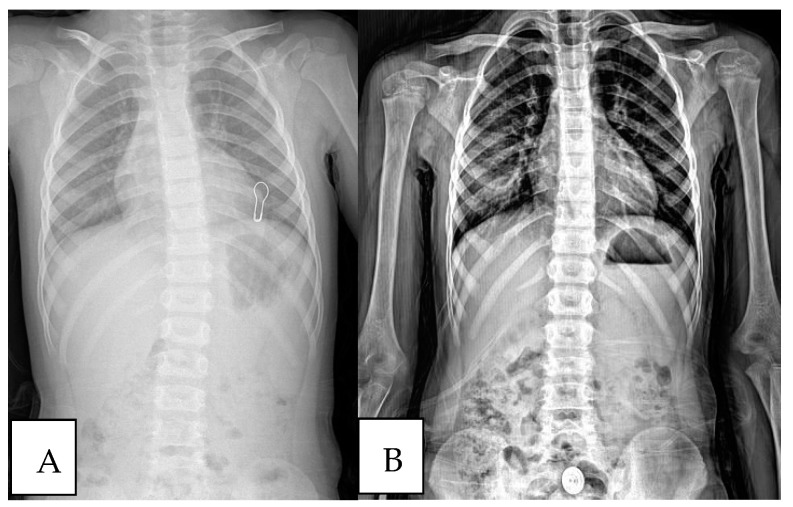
(A) Chest roentgenograph of a patient with a scoliotic attitude detected during preoperative preparation. (B) Post-adenoidectomy scoliosis roentgenograph of the same patient.
